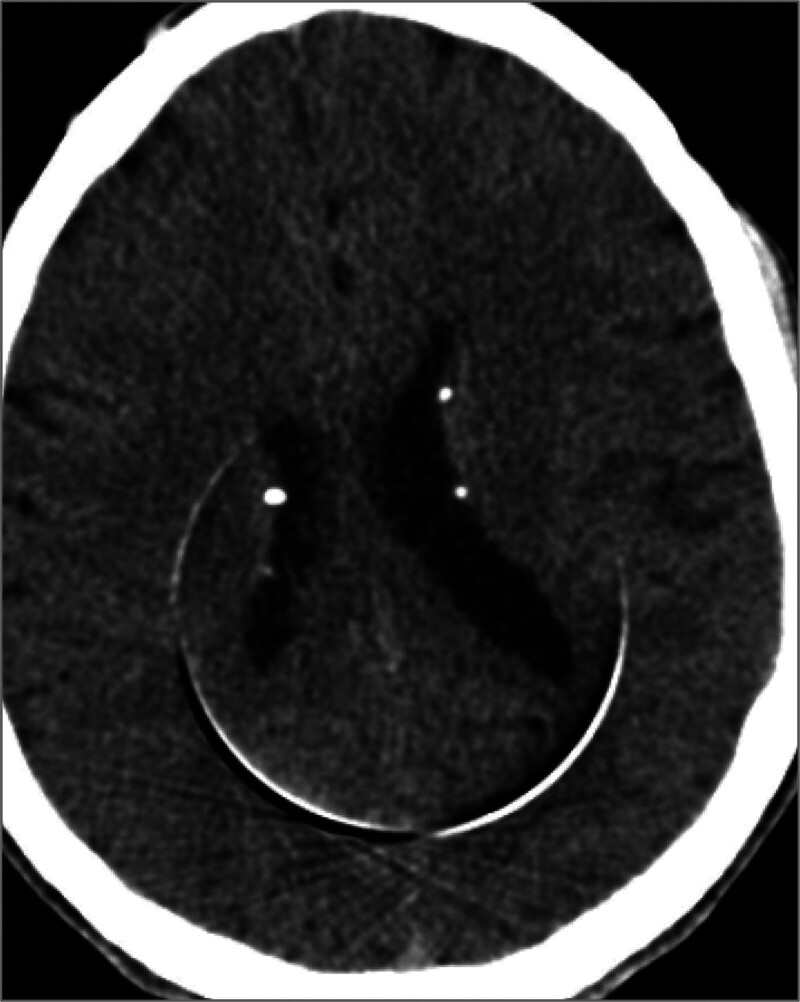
Plain CT scan of the brain (case-1): Multiple calcified subependymal nodules (hamartomas) of tuberous sclerosis.
